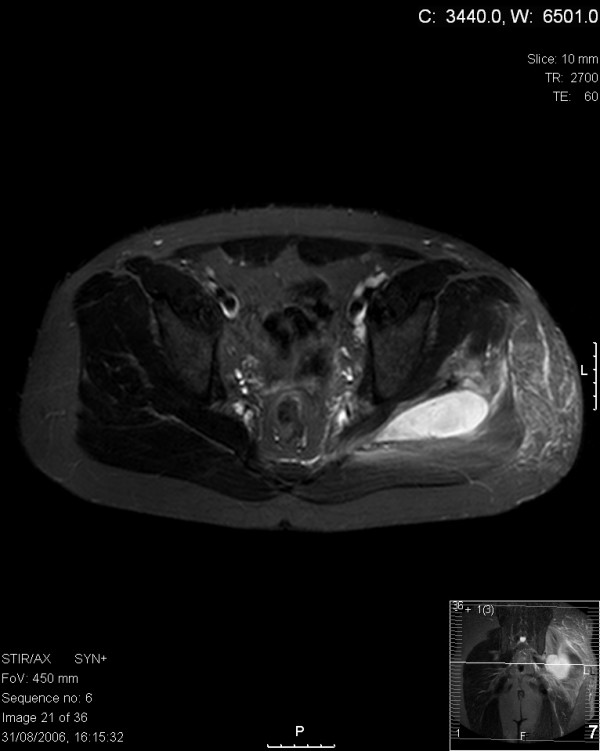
Transverse T2 magnetic resonance imaging section through the hip region showing abscess collection in relation to the sciatic nerve.
